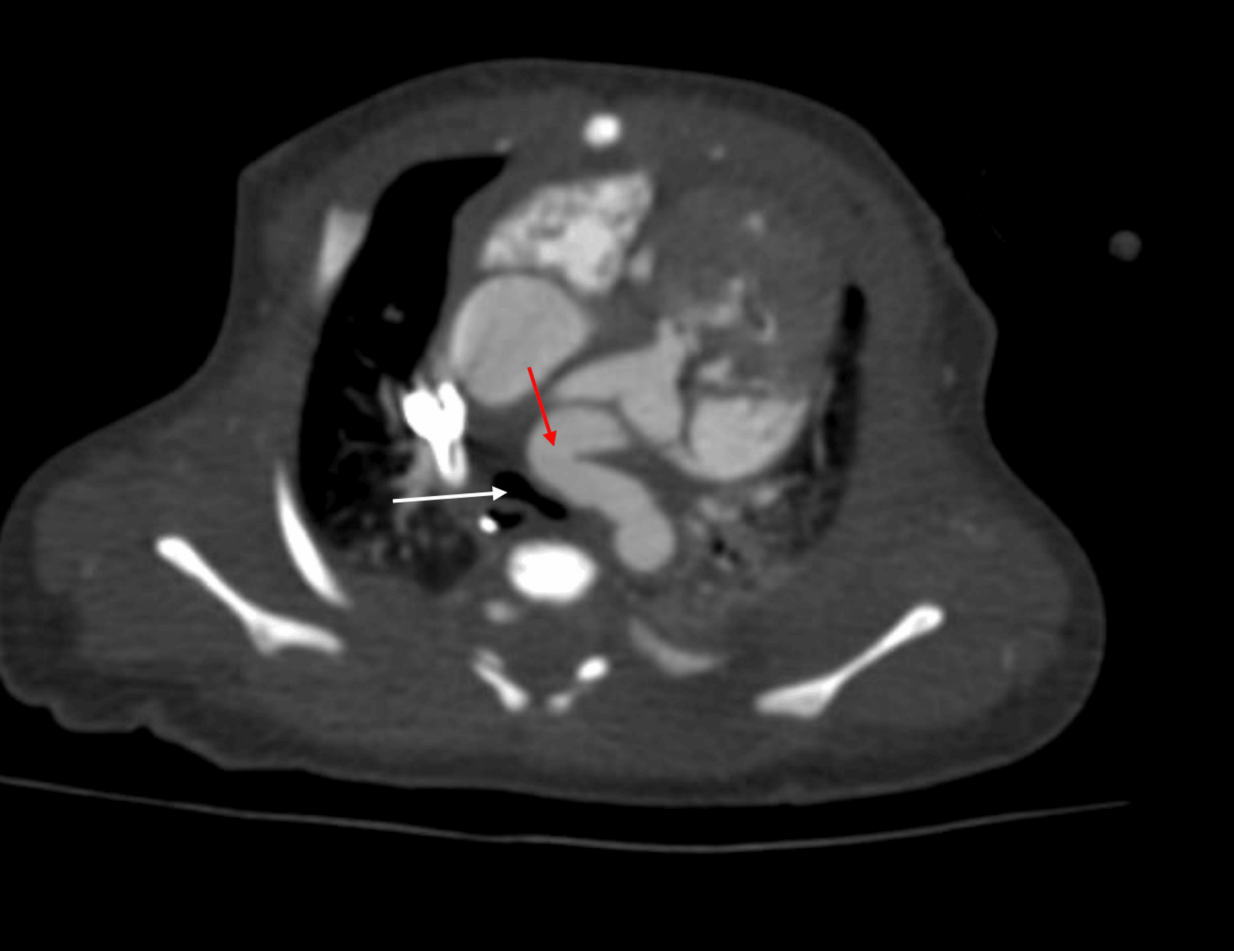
Axial computed tomography image of the enlarged, tortuous patent ductus arteriosus (red arrow) compressing the left main bronchus (white arrow).
